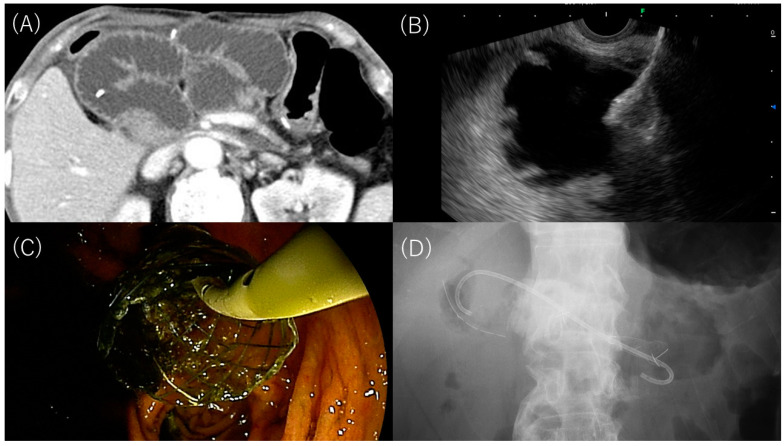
EUS-GJ for malignant afferent syndrome after pancreatoduodenectomy. (A) CT showed the dilated afferent loop. (B) Opening of the proximal end of a dumbbell-shaped FCSEMS. (C) A coaxial DPPS insertion through the FCSEMS. (D) The FCSEMS and DPPS were placed.
